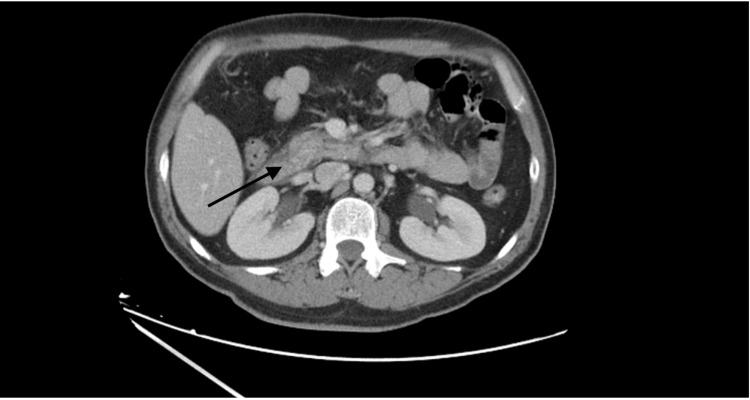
CT of the abdomen revealed pancreatitis of the head of the pancreas with adjacent duodenitis.
